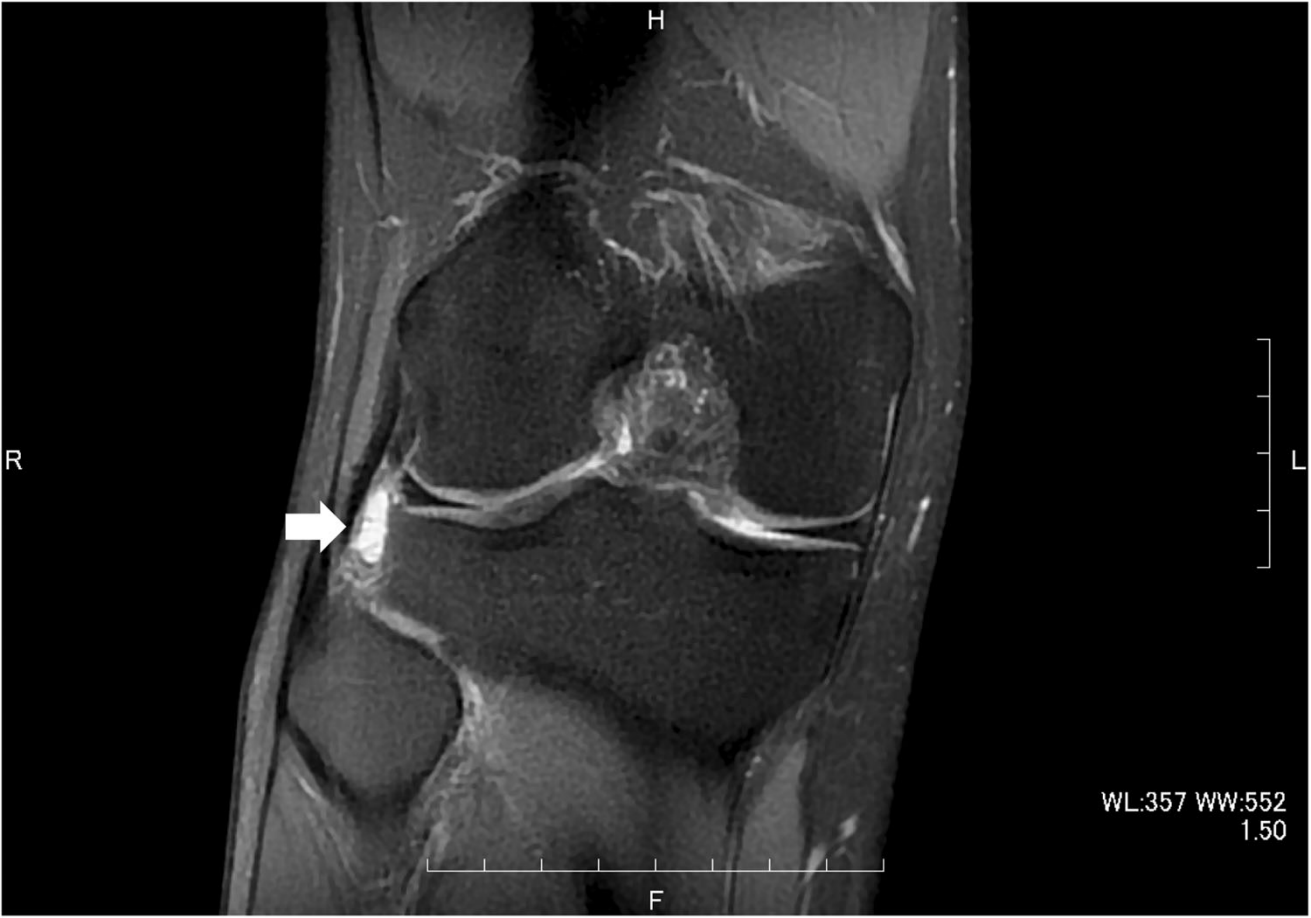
The finding of a post‐operative meniscal cyst (white arrow) in the coronal STIR image of the MRI. MRI, magnetic resonance imaging; STIR, Short Tau Inversion Recovery.
